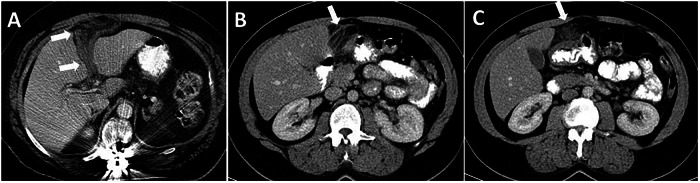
Torsion of the FL in two patients presenting with acute right upper quadrant pain. A Axial plane CT image of a 60-year-old female patient shows a mass-like area in the fissure of the FL with fat attenuation (arrows). B, C Axial plane consecutive CT images of a 35-year-old male patient show fat stranding along the course of the FL (arrows)
